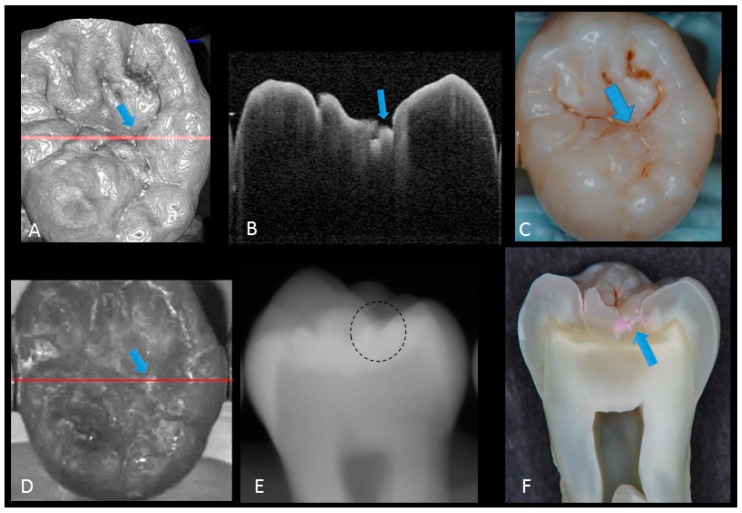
Enamel breakdown: (A) gray-scale three-dimensional (3D) OCT image; (B) cross-sectional OCT image along the red line in (A) and (D). Arrow shows cavitated caries; (C) visual score 2 of enamel cavitation; (D) OCT en face intensity projection; (E) enamel lesion was not distinguished on radiograph due to the superimposition of buccal and lingual cusps; (F) cross-section view with penetration of pink dye enclosed at enamel (score 2). Supplementary Materials: Video 3.
